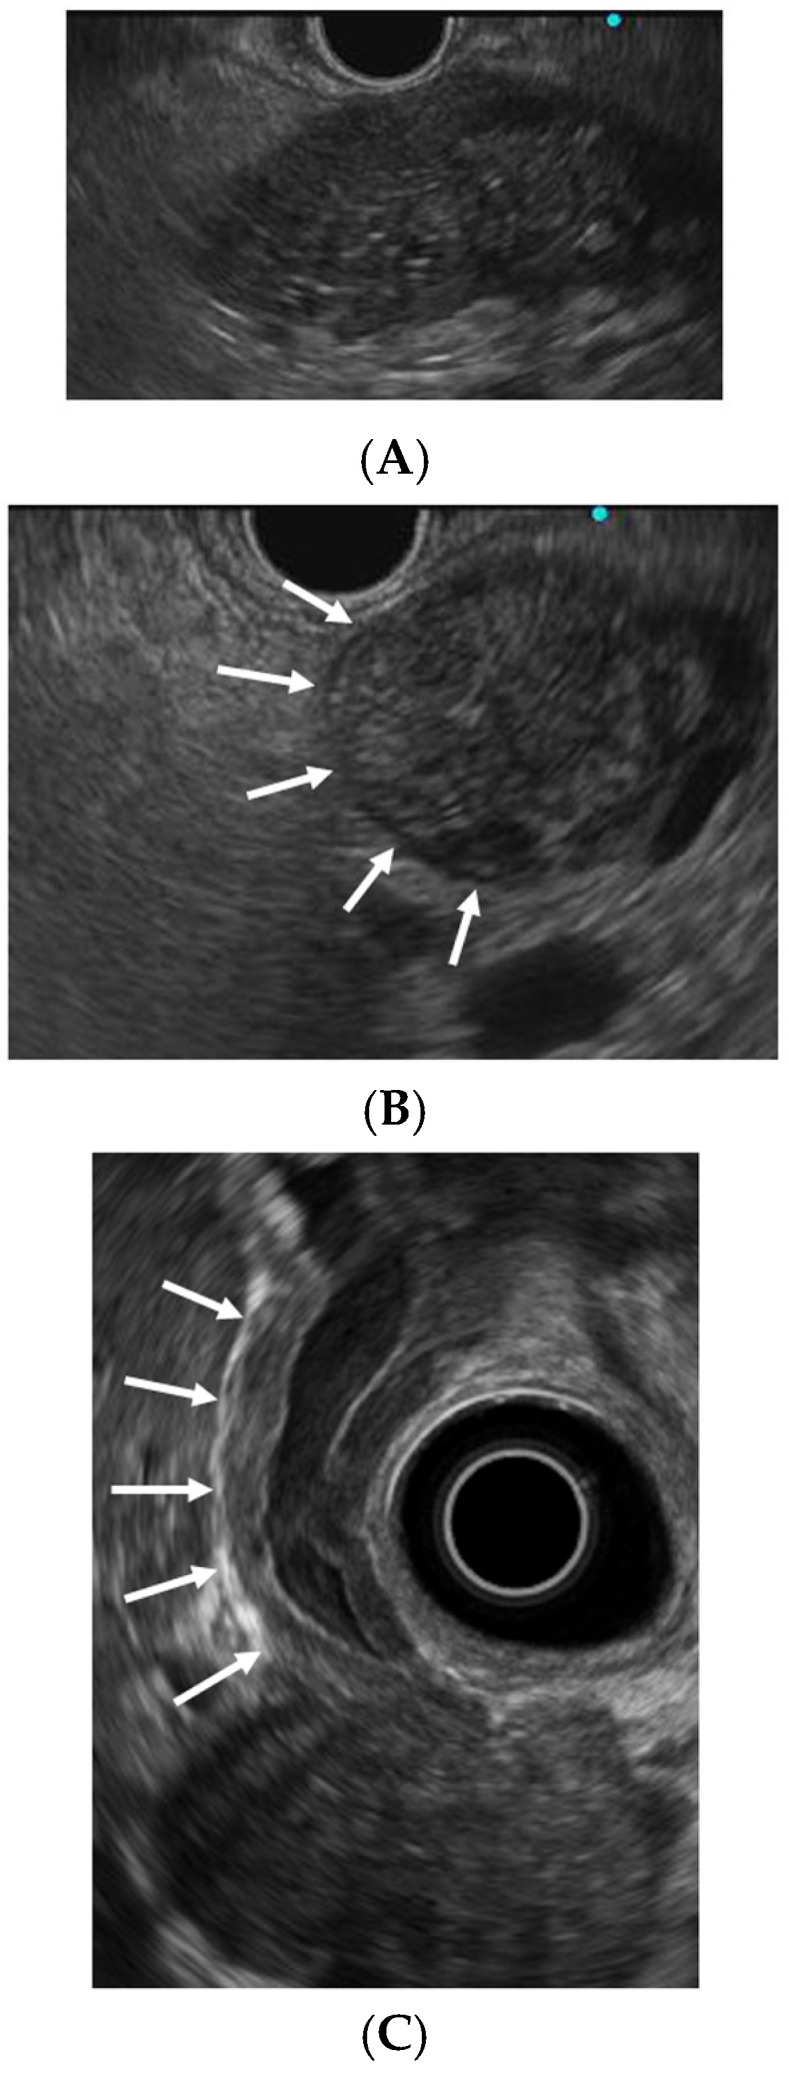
Endoscopic ultrasonographic findings of autoimmune pancreatitis and IgG4-related sclerosing cholangitis. (A) Diffuse pancreatic enlargement with a hypoechoic (“sausage-like”) appearance. (B) Peripancreatic hypoechoic margin (arrows). (C) Bile duct wall thickening (arrows).
